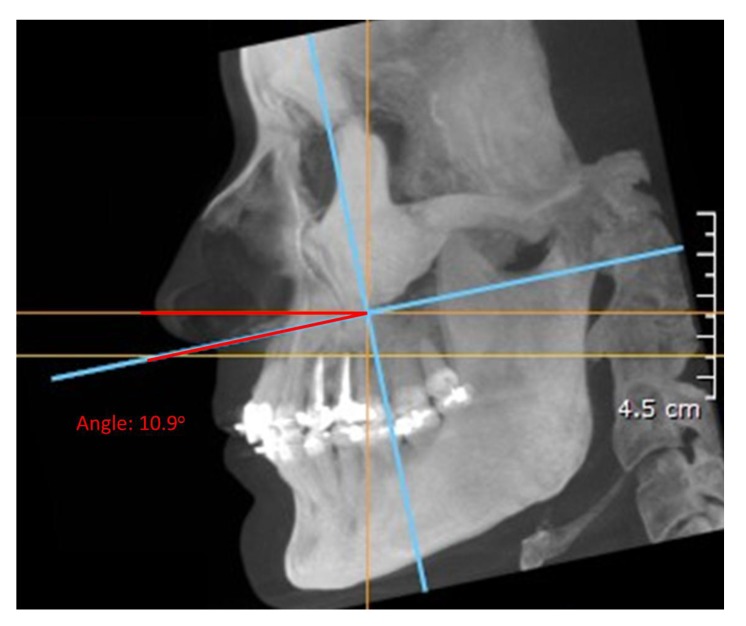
Cephalometric view showing angle between initial and final orientations of CBCT volume.
